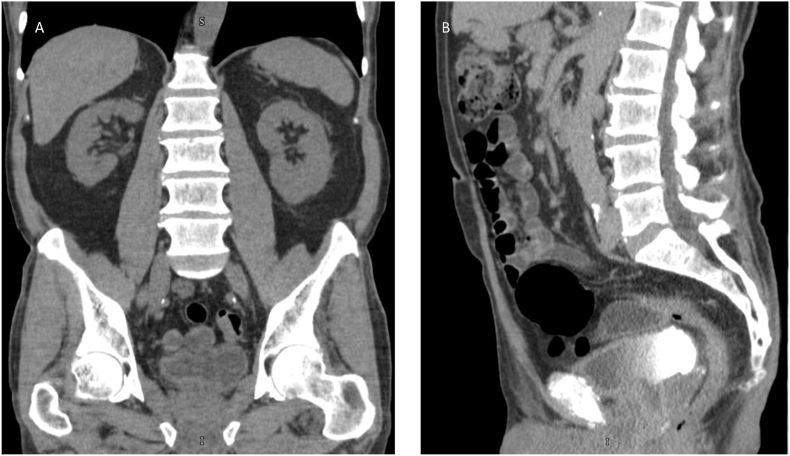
CT urography demonstrating mild concentric bladder wall thickening without discrete lesions or evidence of metastatic disease. (A) Axial contrast-enhanced CT image of the pelvis showing mild concentric thickening of the urinary bladder wall without focal intraluminal lesions. (B) Coronal reformatted contrast-enhanced CT image confirming uniform bladder wall thickening with no radiographic evidence of locally advanced or metastatic disease.
